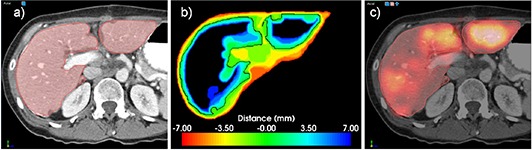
Example of a narrow band registration approach. The liver segmentation (a) is used to build a signed distance narrow band (b) that provides the link of the SPECT gradient with the liver segmentation used in registration. This allows usage of a simple NCC metric in the registration, since its minimum is achieved when the SPECT gradient matches the zero of the narrow band. A typical result is shown in (c), where registration clearly matched SPECT gradients with the liver contour. Overall the method yields reproducible results within 1 mm (shown in Fig. 6).
